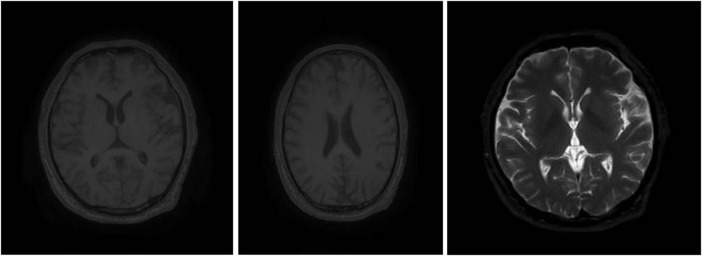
The MRI brain case exposed to occupational methanol inhalation.
